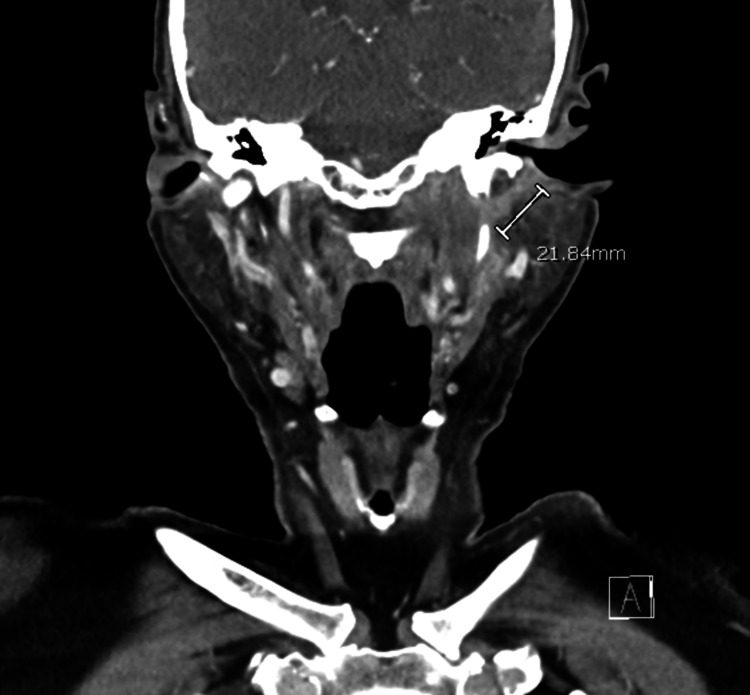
Computed tomography (CT) scan of neck with a coronal view showing hyperenhancing soft tissue along the superior medial deep portion of the left parotid space extending into the left parapharyngeal and carotid space.
